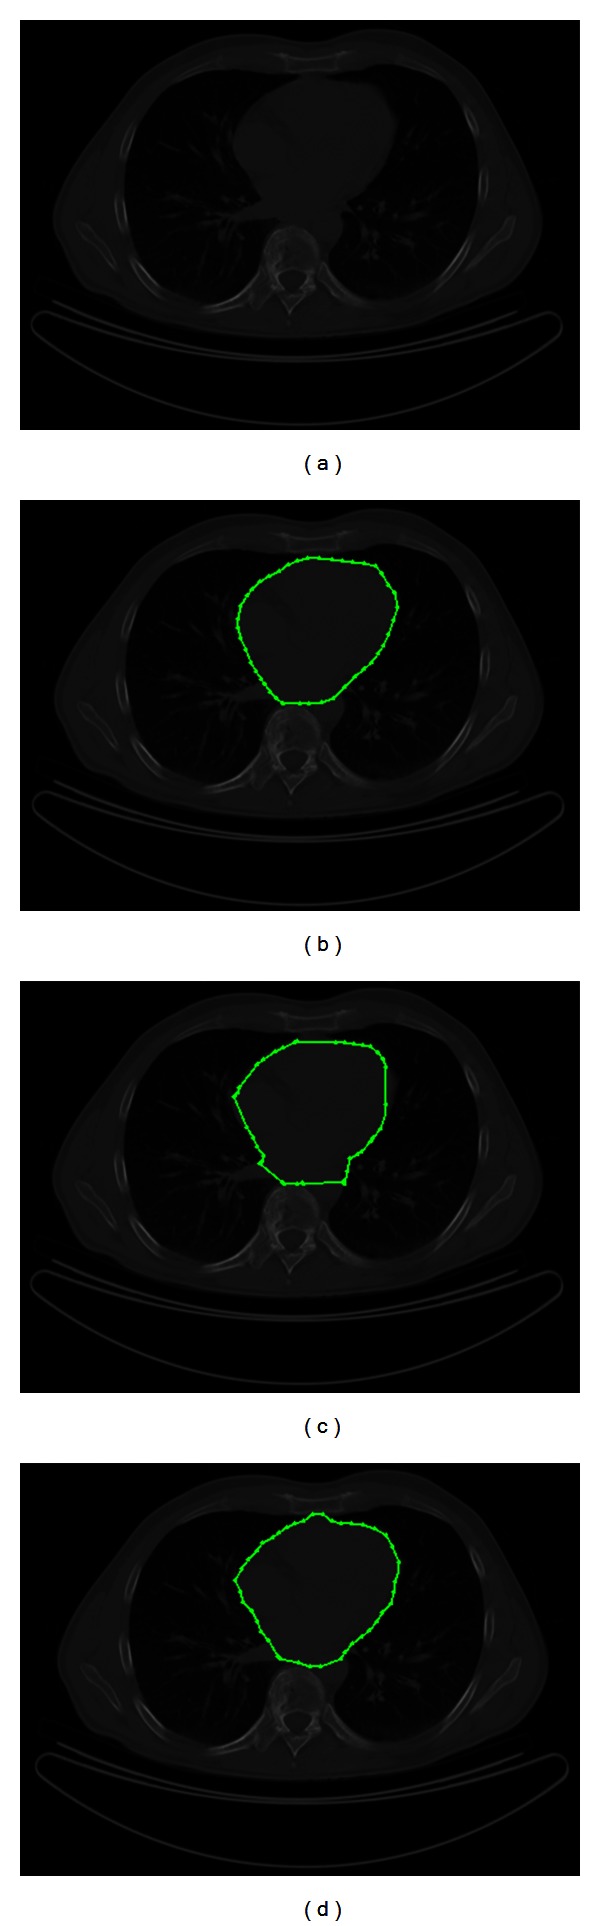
CT image: (a) test image, (b) the human heart outlined by experts, (c) result of traditional ACM and (d) result of MACPSO implementation.
